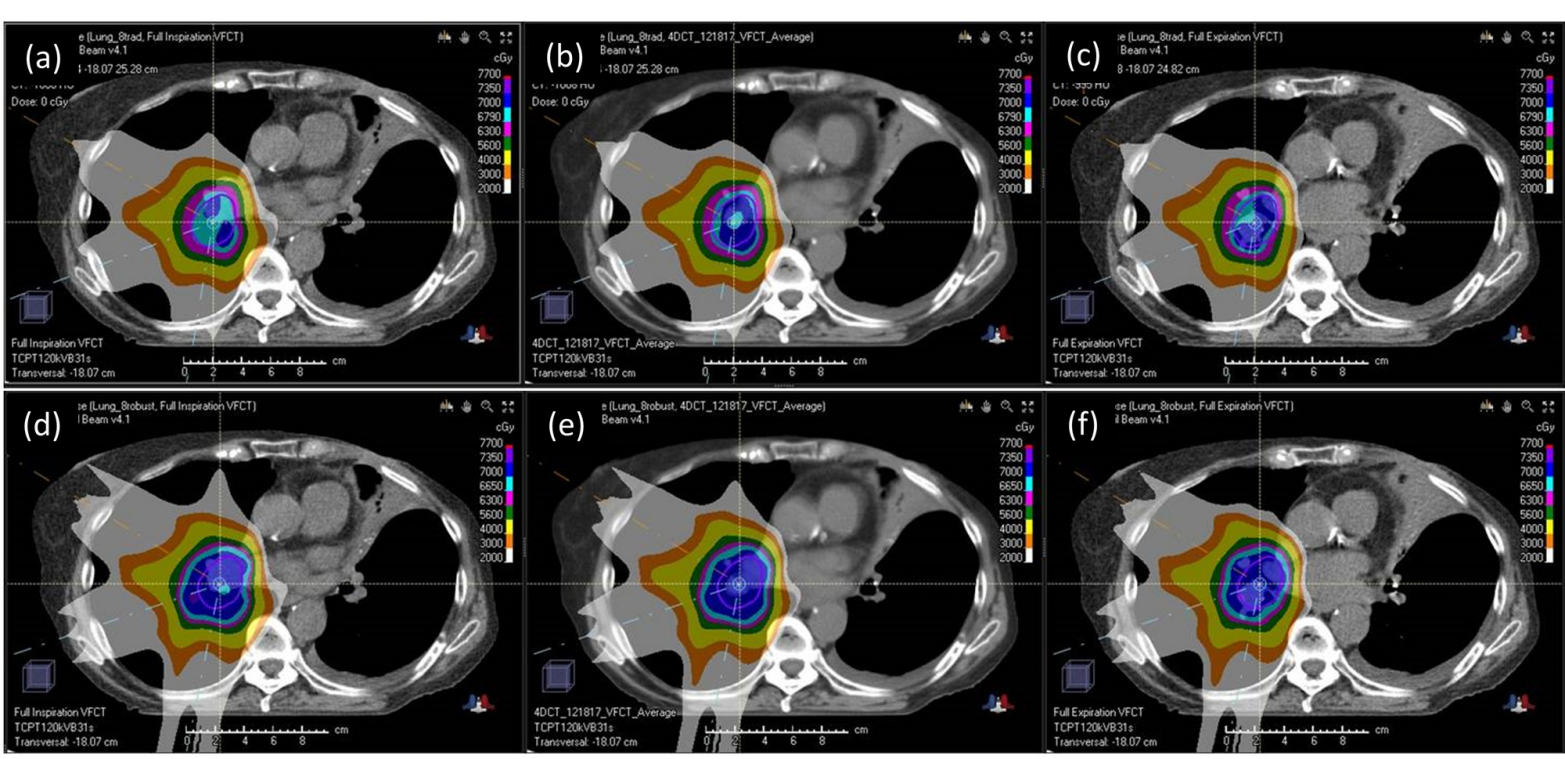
Verification computed tomography: dose distribution Comparison between the 3D optimized plan (a, b, c) and 4D optimized plan (d, e, f), when calculated on the VFCT. The 3D optimized plan is calculated on the VFCT's maximal inspiration phase (a), average (b), and the maximal expiration phase (c). The 4D optimized plan is calculated on the same VFCT scans, i.e., maximal inspiration (d), average (e), and maximal expiration (f). Note that the 3D plan’s target coverage deteriorated on all the VFCT scans, while that of the 4D optimized plan only showed minor degradation. This shows that 4D optimized plans are indeed more robust against inter-fractional variations. This is most likely due to the fact that 4D optimized plans have more stringent robustness criteria when being optimized (details in text). 3D: three-dimensional, 4D; four-dimensional, VFCT: verification computed tomography
